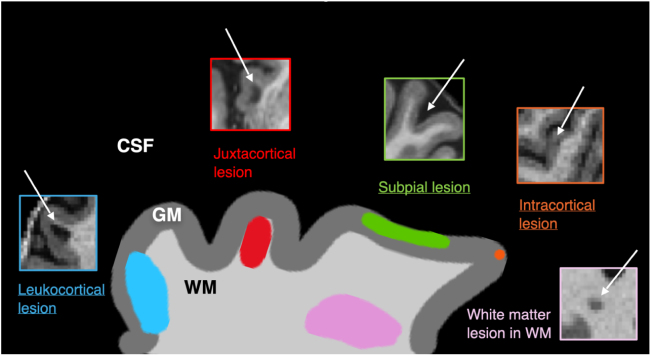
Types of MS lesions categorized by their location with respect to the cortex. Lesions appear on MP2RAGE MRI as hypointense regions (white arrow pointers). CL types are $\underline{\mathrm{underlined}}$, the rest are WMLs. Leukocortical (CL) and juxtacortical (WML) are sometimes difficult to distinguish due to unclear cortical involvement. CSF— erebrospinal fluid, GM—gray matter, WM—white matter.
